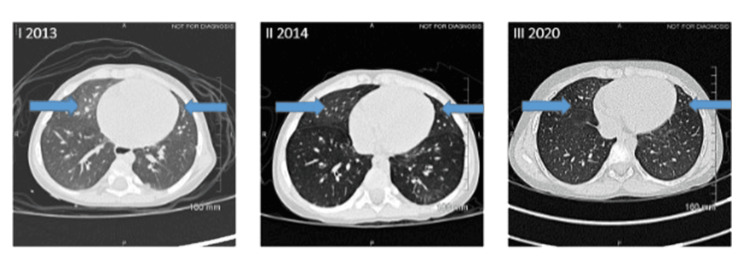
High resolution non-contrast computed tomography of the chest: Thin axial sections show the persistence of ground glass densities in the right middle lobe and lingula in 2013, 2014 and 2020.
